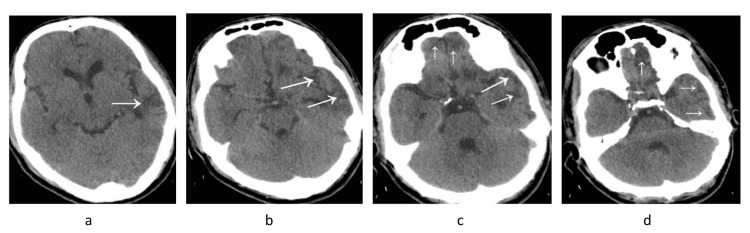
Brain computed tomography scan. Cortical hypodensity in the left temporal lobe (a-d) next to the temporal bone and around the Sylvian fissure of the same side (b) as well as at the inferior aspect of the frontal lobes bilaterally (c, d).
